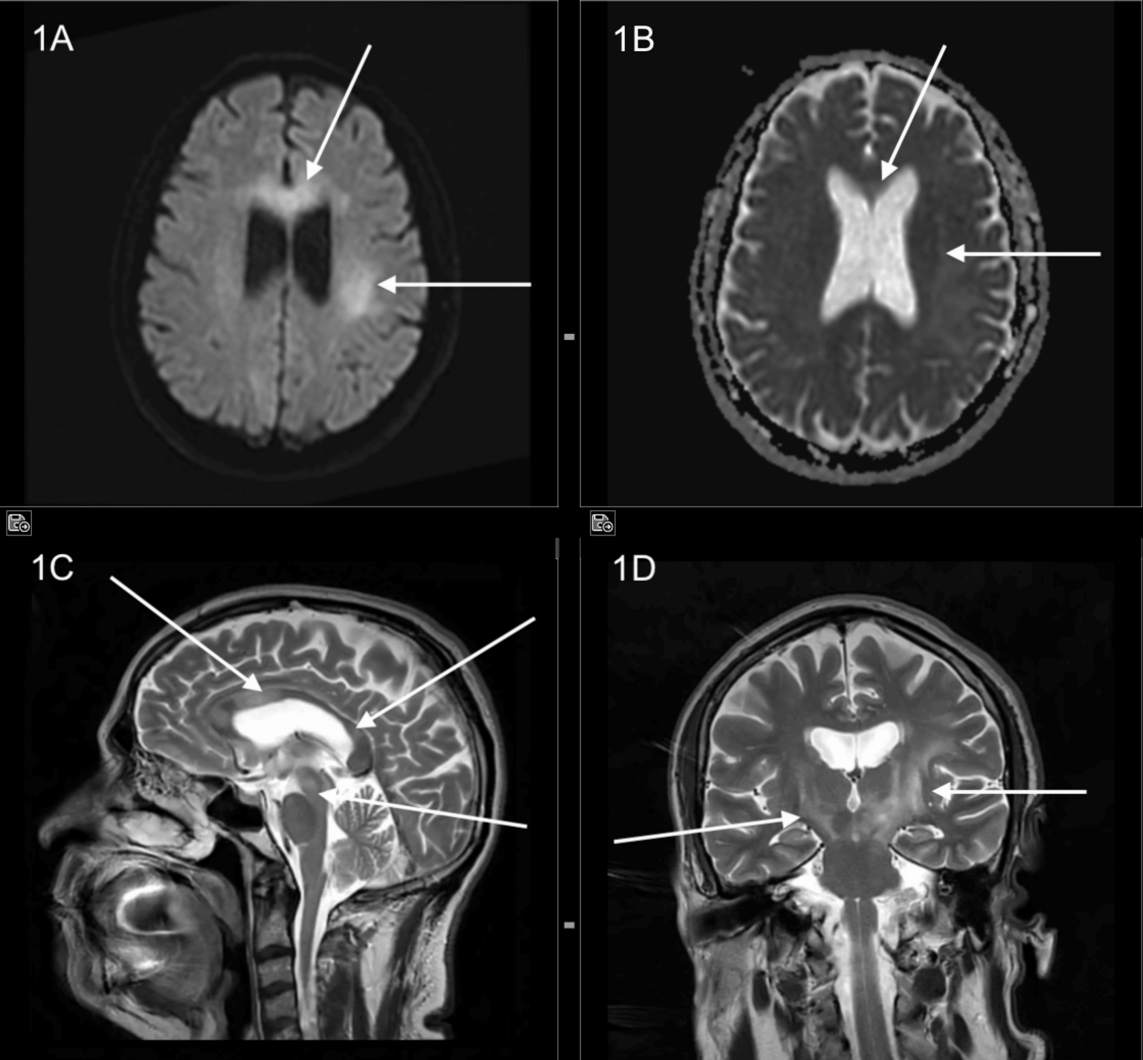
(A, B) DWI and ADC images showing DWI hyperintensity with mild reversal on ADC-weighted images in periventricular white matter and corpus callosum. (C, D) T2 sagittal (C) and T2 coronal (D): Multiple lesions showing heterogeneous signal intensity with hyperintense oedema involving corpus callosum, gangliocapsular regions, bilateral periventricular white matter, brainstem and cerebral peduncles. DWI: diffusion-weighted imaging; ADC: apparent diffusion coefficient.
